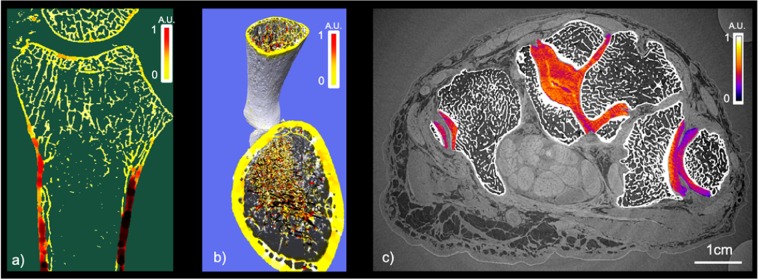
Bone and cartilage quality evaluation: (a) Bone quality of an ulna and radius bone: false color representations of the local thickness. (b) Estimation of the rode plate distribution in the trabecular bone with false color representation. (c) PCI-CT axial slice with a color map of the cartilage density in false color.
